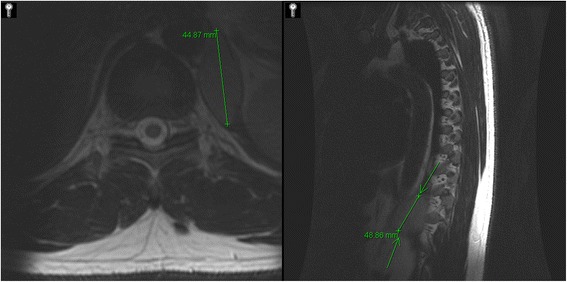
Axial and sagittal slices of MRI thoracic spine without contrast denoting a 5 cm × 4.5 cm ovoid hyperintense soft tissue structure medially at left lung base possible a bronchogenic cyst
